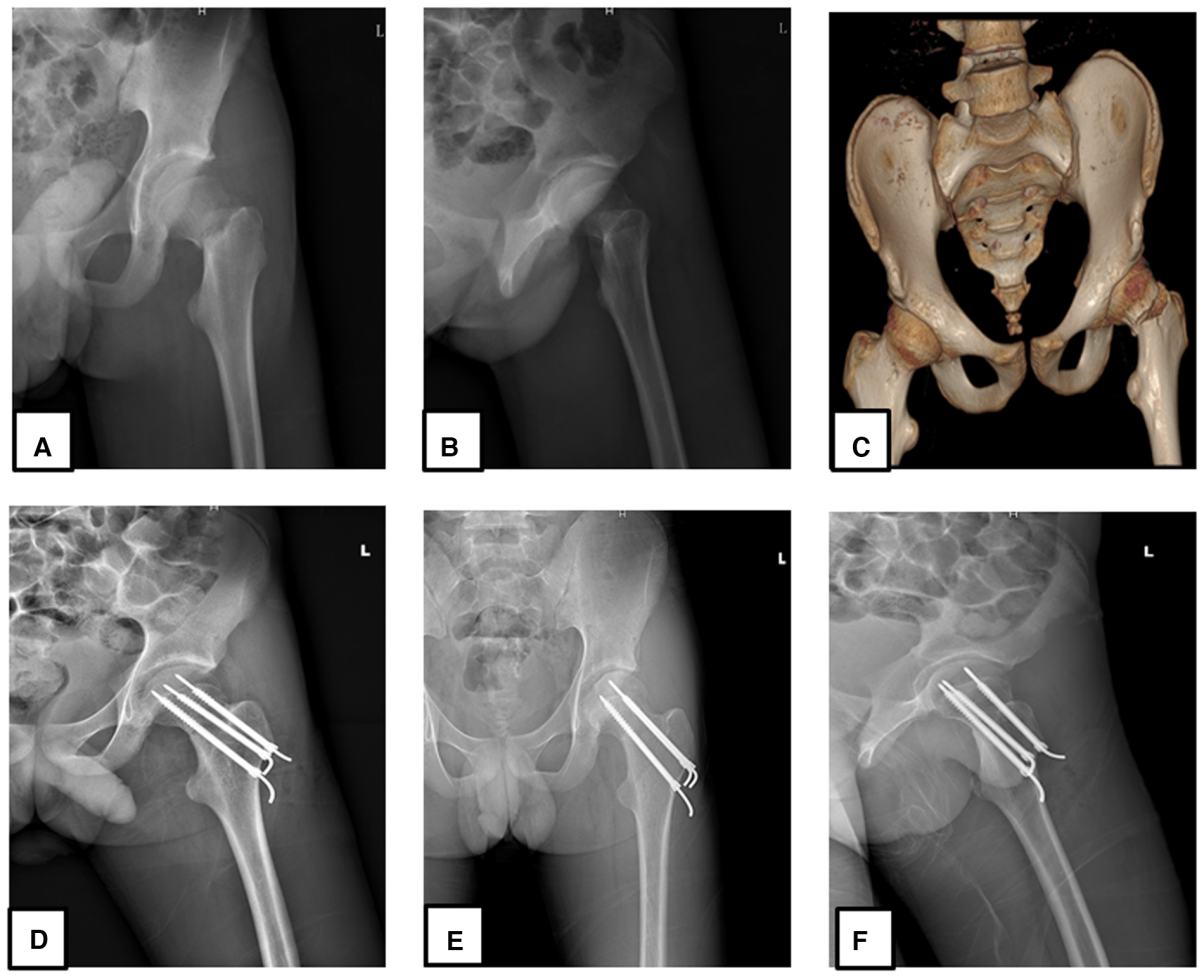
A 14-year-old male patient who sustained a Delbet type II femoral neck fracture after a fall, treated with cannulated cancellous screw combined with K-wire 24 h after injury. Immediate preoperative x-ray anteroposterior view (A), lateral view (B), 3D CT scan anteroposterior view (C), immediate postoperative x-ray anteroposterior view (D,F), and lateral view (E) were done.
